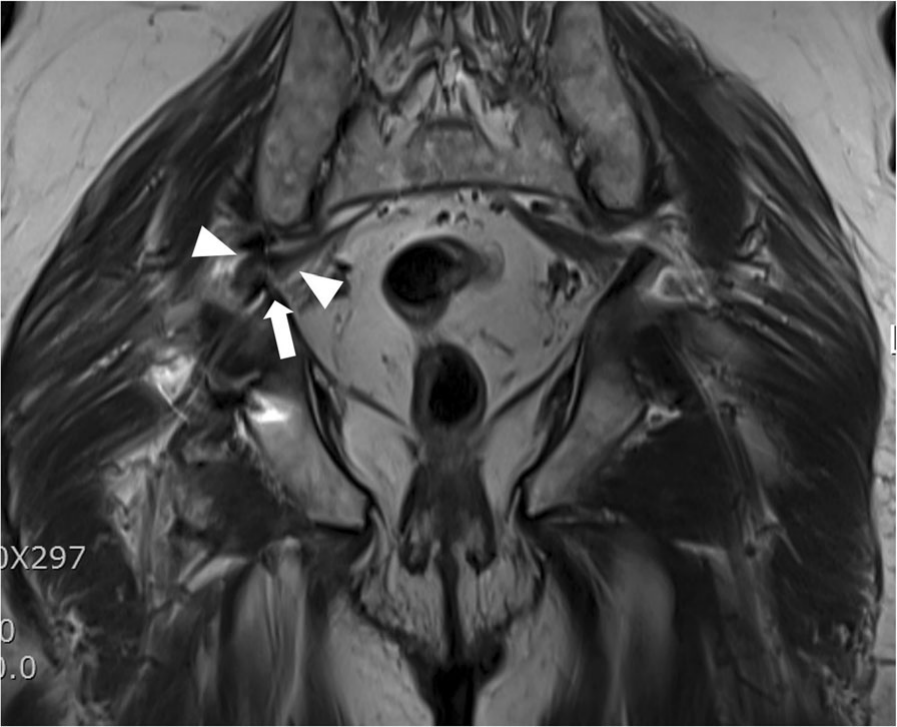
T2 turbo spin echo magnetic resonance image displaying a screw protrusion (arrowhead) and irritated piriformis muscle (arrow)
